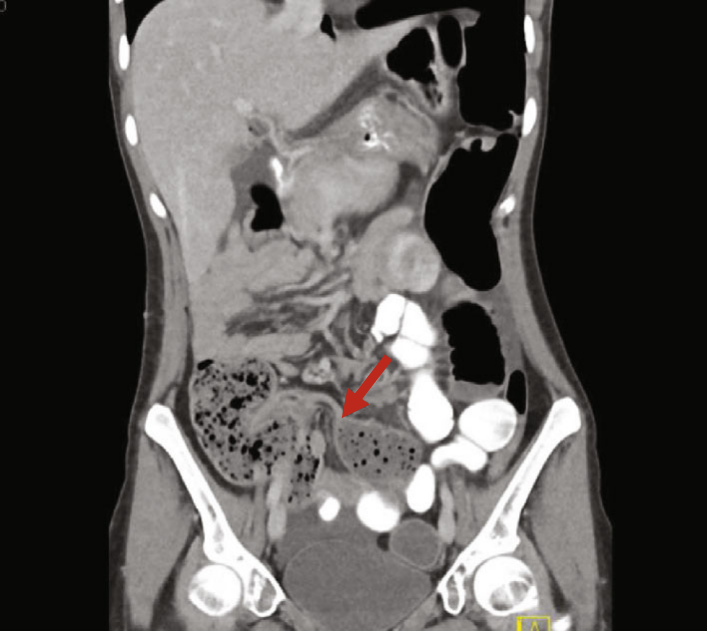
CT of the abdomen and pelvis showing with the red arrow indicating a transition point just proximal to the terminal ileum.
